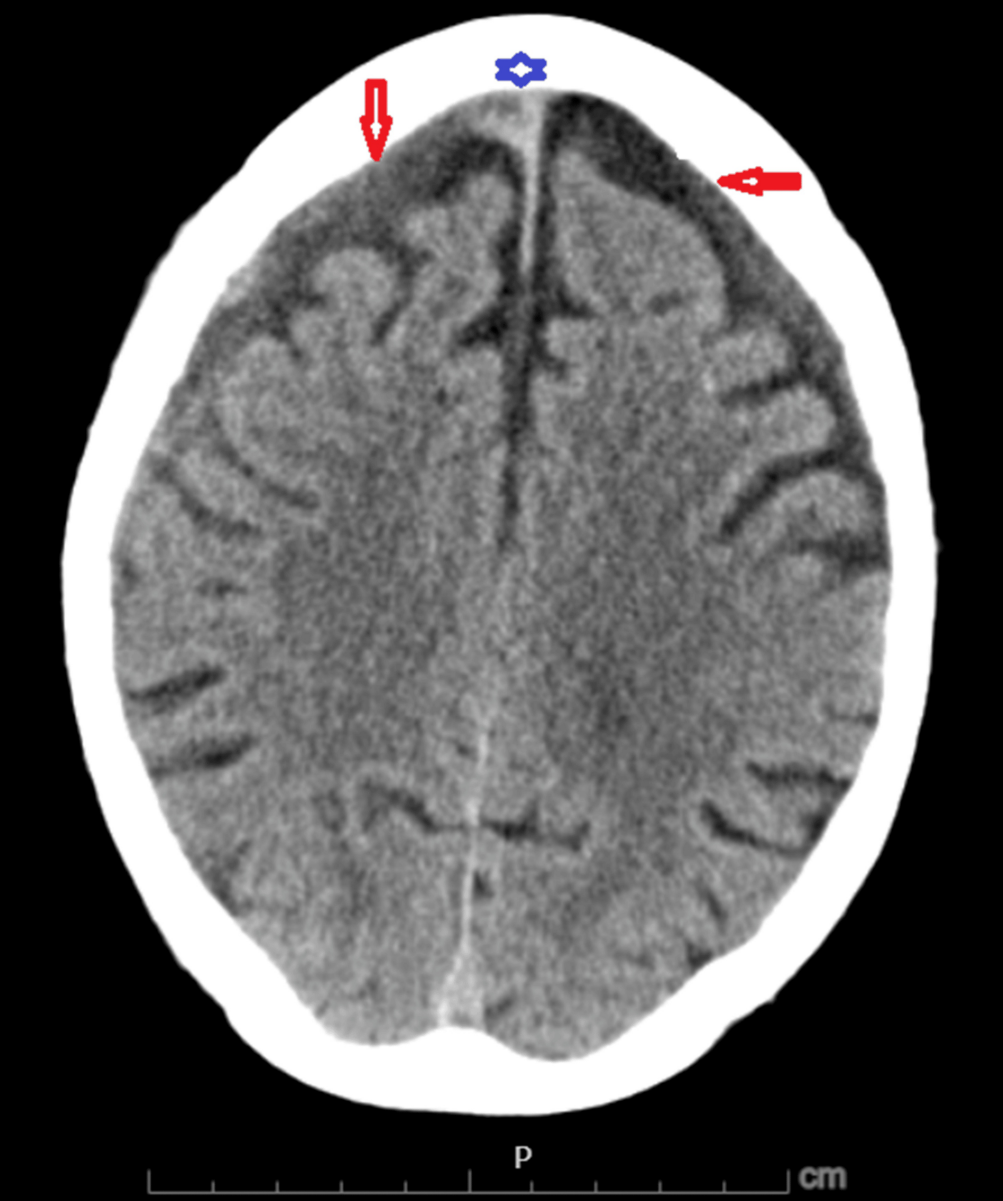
Non-contrast head CT showing acute-on-chronic bilateral subdural hematomas Axial non-contrast CT of the head demonstrating bilateral subdural hematomas. Chronic components are indicated by red arrows, while acute hyperdense components on the right are marked with a blue star. The maximal thickness measures 11 mm on the right and seven mm on the left. There is no significant midline shift or hydrocephalus.
